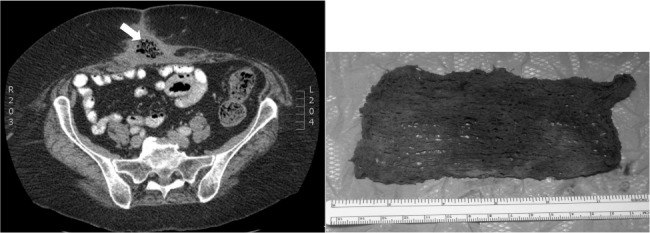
An obese patient developed a wound infection at the site of an incisional hernia repair performed 10 weeks previously. This was treated in the community with negative pressure wound therapy (NPWT). Foul-smelling discharging pus persisted from a wound sinus after cessation of NPWT. Computed tomography (left) showed an area of inflammation with multiple air pockets (arrow) in the subcutaneous tissue of the anterior abdominal wall. Wound exploration under general anaesthesia revealed a sponge (without a radiopaque marker) used for the NPWT dressings in the subcutaneous fat (right). The sponge was removed, the wound healed by secondary intention and the patient made an uneventful recovery.
